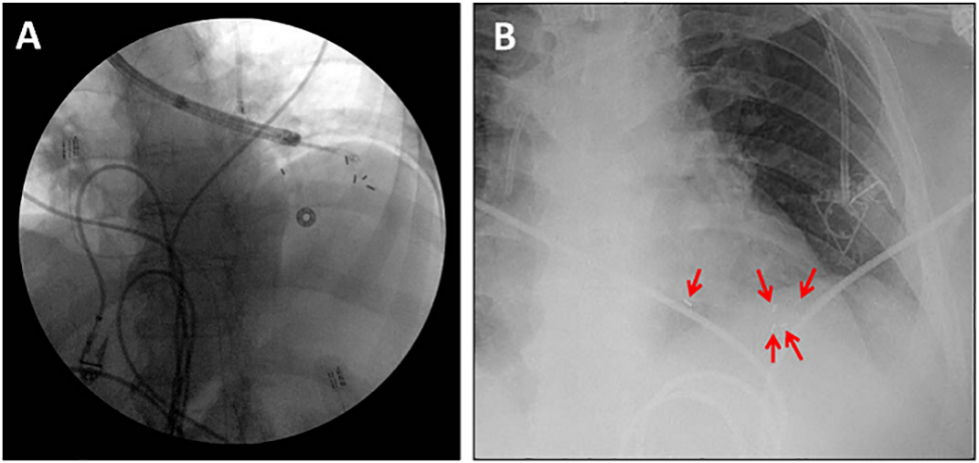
SuperLock nitinol coil fiducials deployed into the left lower lobe of the lung through electromagnetic navigation bronchoscopy. Five fiducials were deployed, four in or within 1–2 cm of the tumor, and one further away as visualized during the placement procedure (panel A), and at a post-procedure chest radiograph (panel B). The fiducials were centered around the tumor, which is not well-visualized in the kV images.
